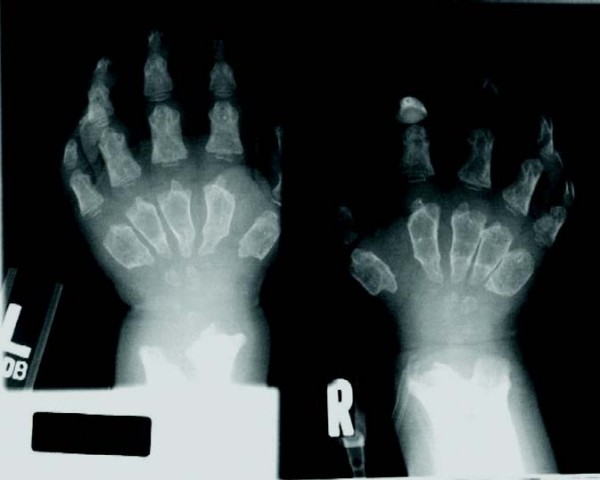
Rapidly progressing 3-year old male MPS VI patient: radiograph of hand.
